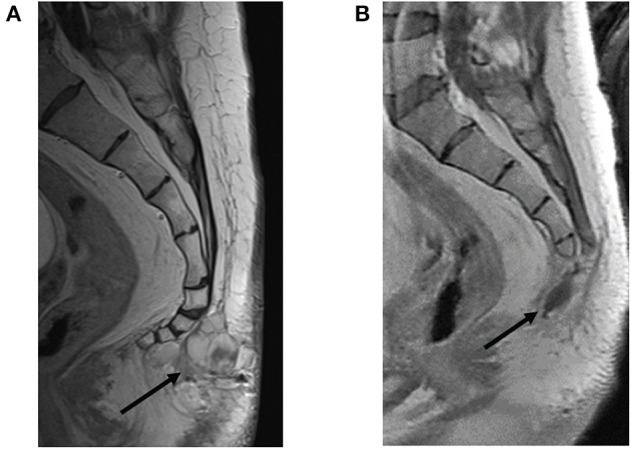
Restaging of recurrent soft tissue mass prior to and following resection. (A) Pre-resection sagittal view with contrast-enhanced T1-weighted image showing a multilobulated mass extending from the first through fourth coccygeal segments bordered by a thin plane of fat interposed between the tumor and coccyx without evidence of coccygeal invasion (black arrow). (B) Status post-coccygectomy and resection cavity (black arrow) of the previously described associated lobulated mass.
